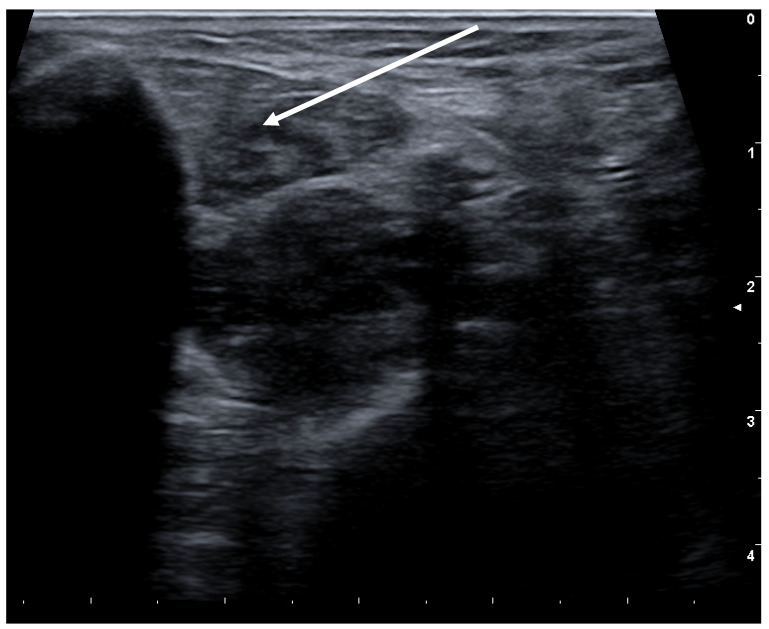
Sonography of the calf with the long toe flexor (M. flexor digitorum longus).
